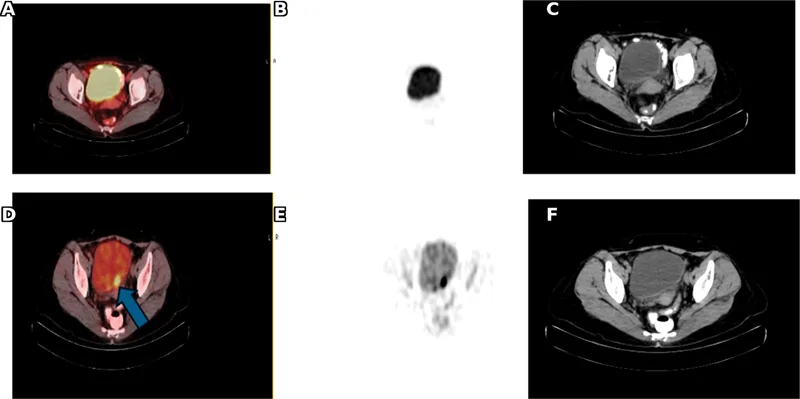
Representative case of nonmuscle-invasive bladder cancer (BC; Ta) on diuretic-enhanced dual-phase F-18 FDG PET/CT. (
